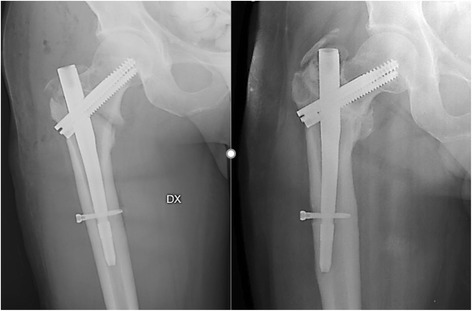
Radiographs of InterTan nail with cephalic screw with TAD greater than 35 mm during surgery and after 5 months with valgus mobilisation of the intramedullary nail for a fracture 31-A1
